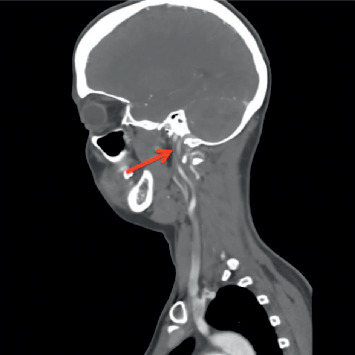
CTA shows a short segment of high-grade narrowing of the cervical right vertebral artery at the C2-3 level.
